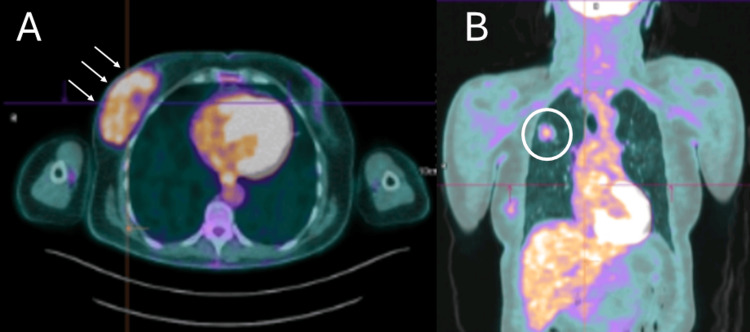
A fluorodeoxyglucose (FDG)-positron emission tomography (PET) scan showing a moderate uptake of 18F-FDG (SUVmax = 3.15) in a large formation in the outer quadrants of the right breast (white arrows), and a moderate uptake of 18F-FDG (SUVmax = 2.35) in a pulmonary lesion of the right upper lobe (white circle).
